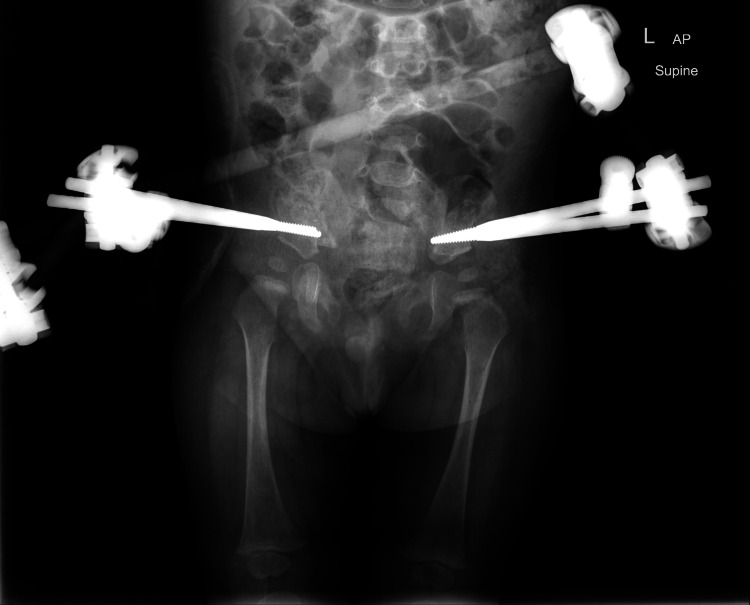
Postoperative plain radiograph. The image shows healed osteotomies with the pins not penetrating the hip joints.
